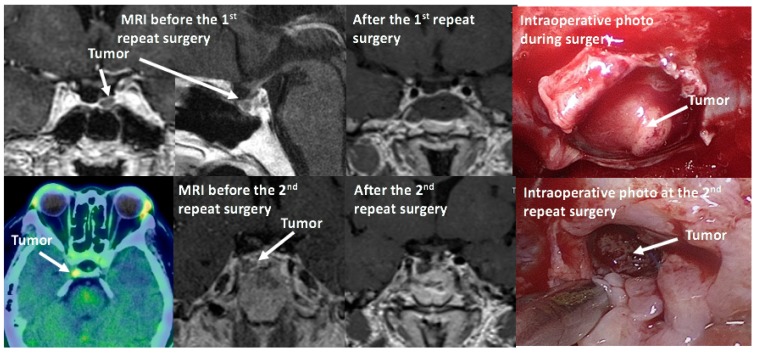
This 52-year-old lady had undergone transsphenoidal surgery for the treatment of pituitary apoplexy (tumor was large macro-tumor at that time). Several years later she showed Cushing’s syndrome and a detailed examination confirmed Cushing’s disease due to pituitary tumor located in the left side of pituitary which was removed by the first repeat surgery. However, both ACTH (91.3 pg/mL) and cortisol level (13.8 μg/dL) were still high. 11C-methionine PET-CT confirmed active small tumor still remained at the right posterosuperior sellar region which had been separated in the primary surgery and was removed by the 2nd repeat surgery (ACTH and cortisol levels reduced to 7.4 pg/mL and 2.6 μg/dL, respectively).
